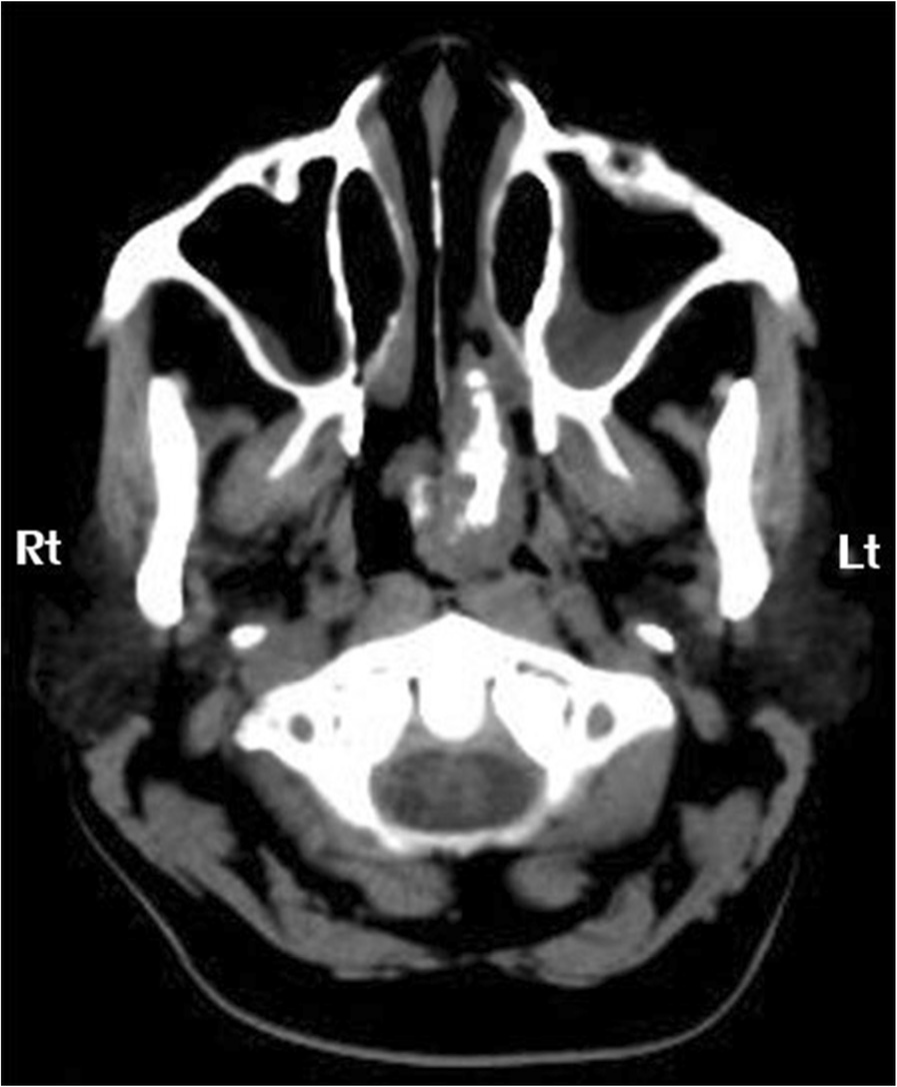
Axial view computed tomography soft tissue window demonstrated a 5×3×3 cm lobulated non-enhancing mass in posterior nasal cavity and nasopharynx arising from the left posterior nasal septum. Dense central calcification is noted. *Lt* left, *Rt* right
